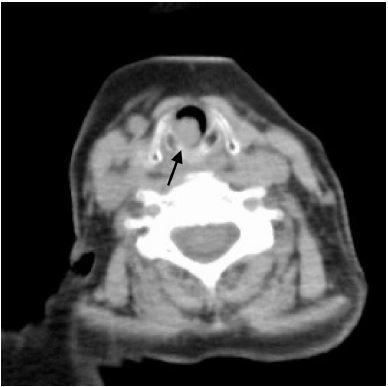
A chest computed tomography showing the endotracheal mass (arrow) immediately below the glottis.
